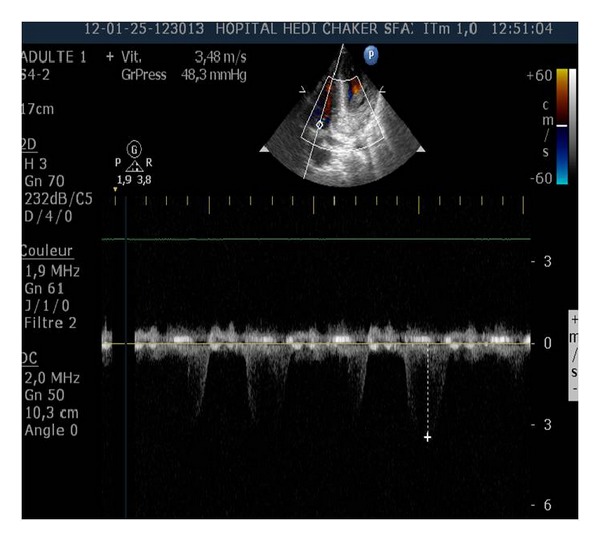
Systolicpulmonary artery pressure (SPAP). Continuous-wave Doppler echocardiography was used to estimate SPAP.
